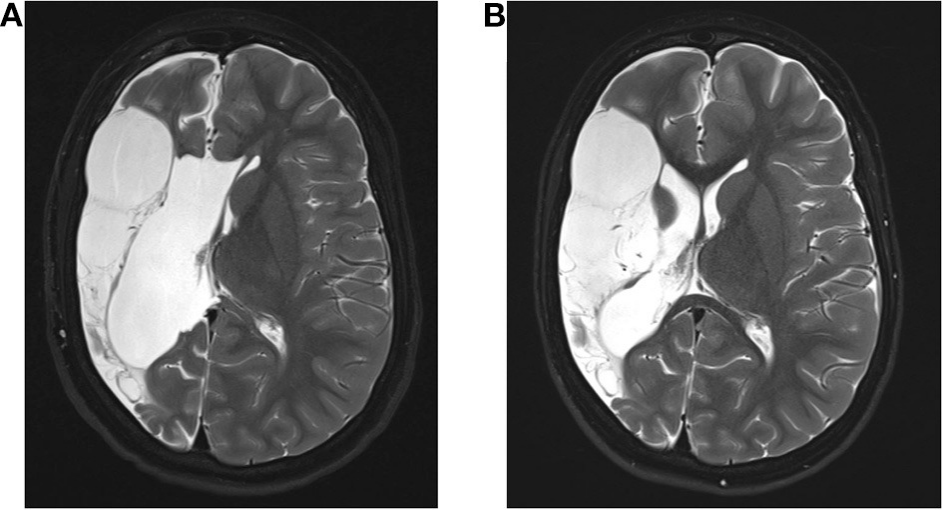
Magnetic resonance imaging (MRI) of the patient. (A) Shown is the preoperative MRI of the 2; 10 year old patient. (B) Normal MRI of the patient 6 months after surgery showing the malacic transformed medial infarct on the right side with no evidence of hemorrhage, infarction, or liquor circulatory disturbances.
